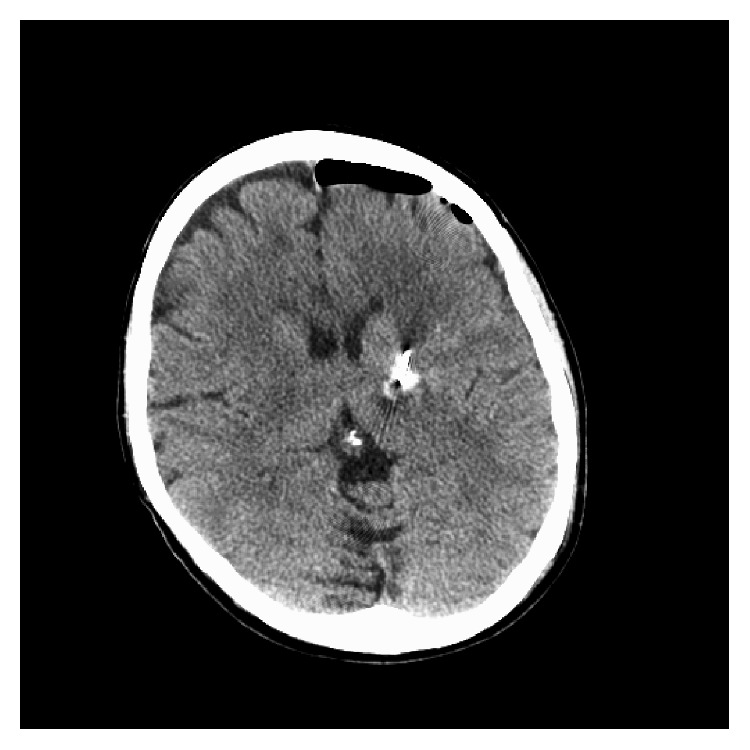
CT head demonstrated a small hemorrhage at the tip of the lead.
